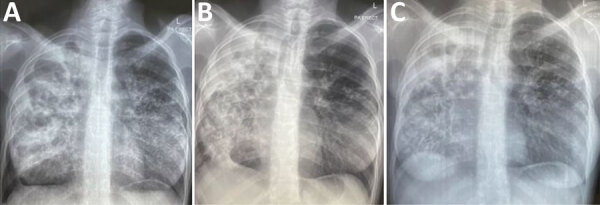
Chest radiographs showing lungs of patient in Namibia who developed bedaquiline resistance. A) At treatment initiation; B) at culture conversion; C) 3 months after conversion.
